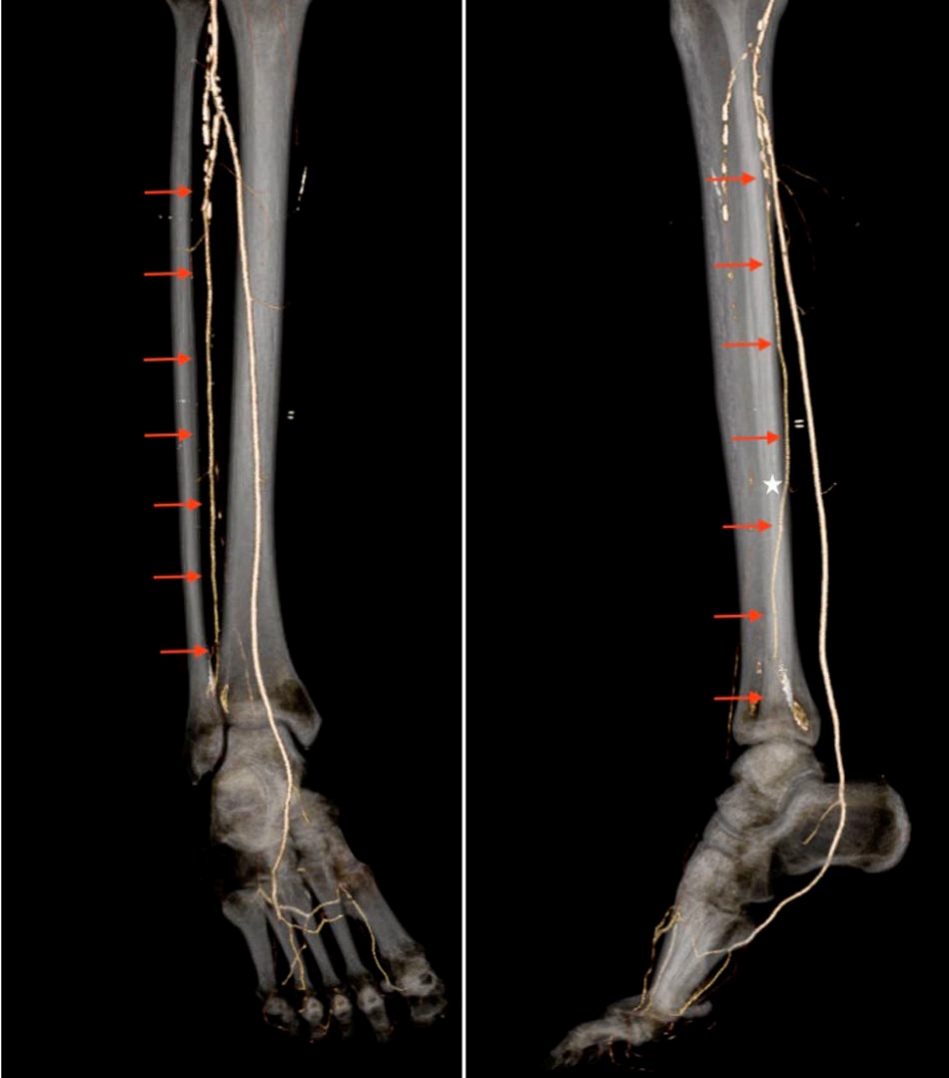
Three-dimensional reconstructions of the computed tomography angiogram with the peroneal artery highlighted by red arrows. Notice the point at which is crosses anteriorly into the syndesmotic plane (white star). [See online article for color figure.]
